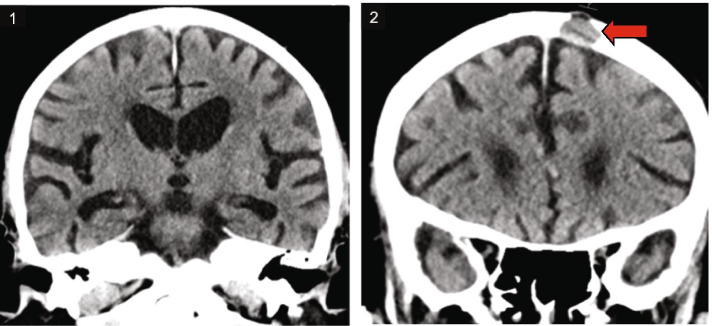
Brain CT images showing white matter changes Fazekas 2, cortical atrophy GCA1-2, medial temporal lobe atrophy with reduced hippocampus, MTL 3 (1) as well as a single lytic metastasis within the left side of the frontal bone (red arrow) (2)
